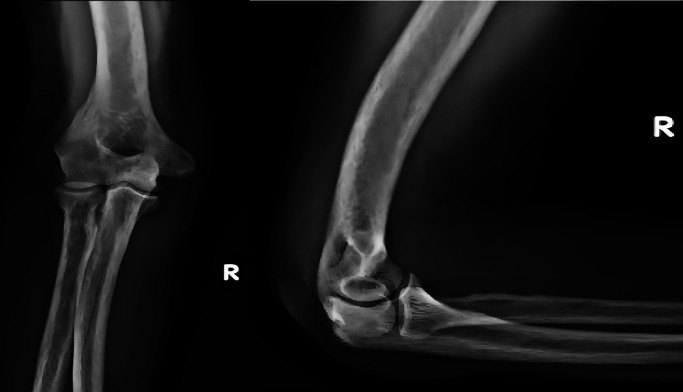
Anteroposterior and lateral radiographs of Case 2 with avulsed triceps tendon.
